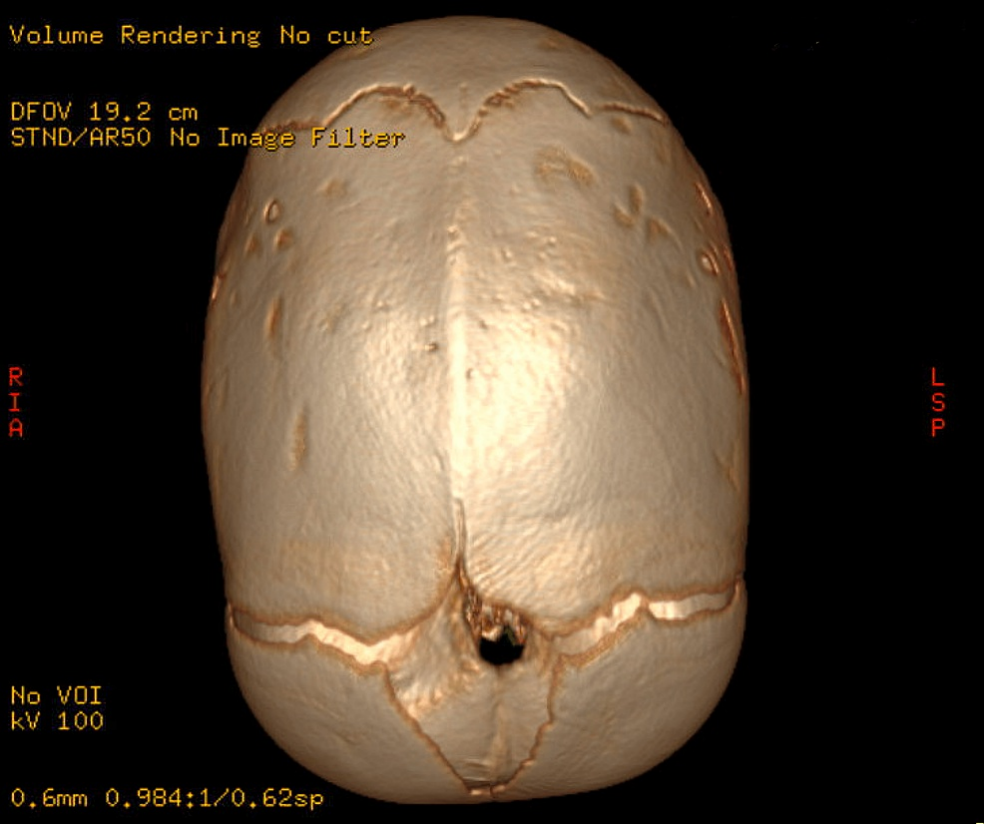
CT of the head showing sagittal synostosis.
